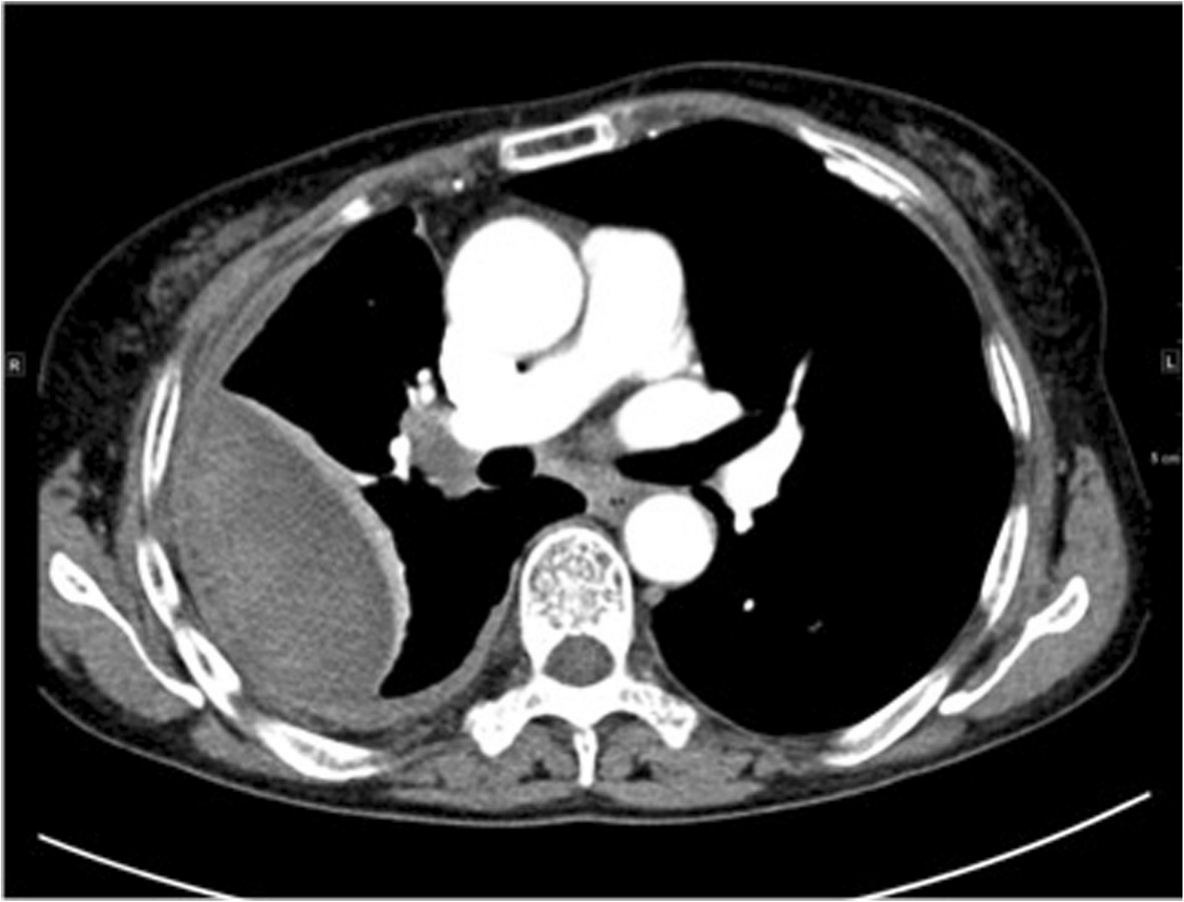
Chest CTA showed encapsulated pleural effusion with atelectasis on the right side, multiple enlarged lymph nodes could be seen in right intercostal, right hilar and mediastinal area
